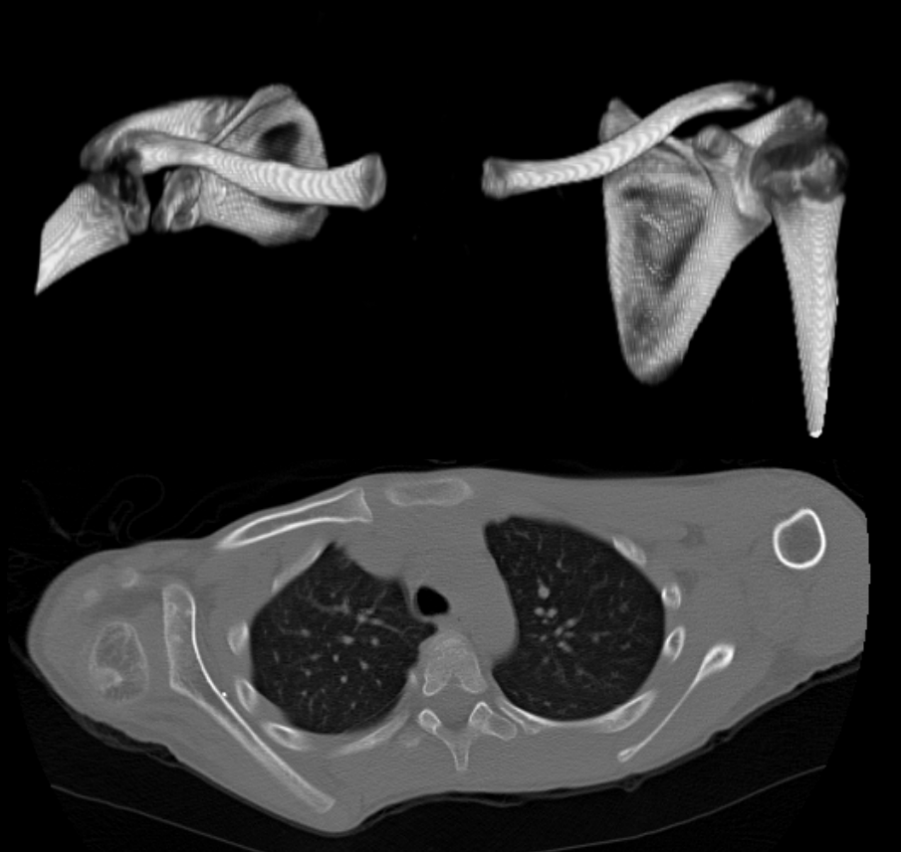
CT images showing SHEAR deformity present after humeral osteotomy. Ten year old boy after unsuccessful humeral osteotomy with right-sided SHEAR deformity demonstrated in 3D CT anterior view (above) and posterior subluxation demonstrated in axial view (below).
